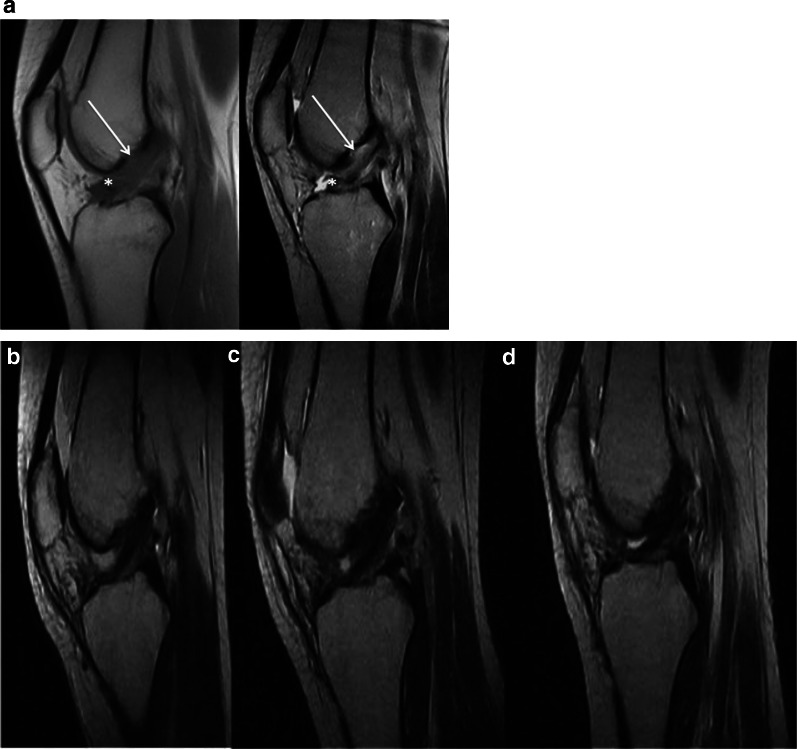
Pre- and postoperative images of patient #3. a Sagittal preoperative MRI T1-TSE (left) and T2-TSE (right). Tear location (arrow): type III; tissue quality (asterisk): good b Sagittal T2-TSE 1 month post-op. Morphology: grade 1 (normal); signal intensity: grade 1 (isointense) c Sagittal T2-TSE 3 months post-op. Morphology: grade 1 (normal); signal intensity: grade 1 (isointense)  d Sagittal T2-TSE 6 months post-op. Morphology: grade 1 (normal); signal intensity: grade 1 (isointense) *TSE* turbo spin-echo
